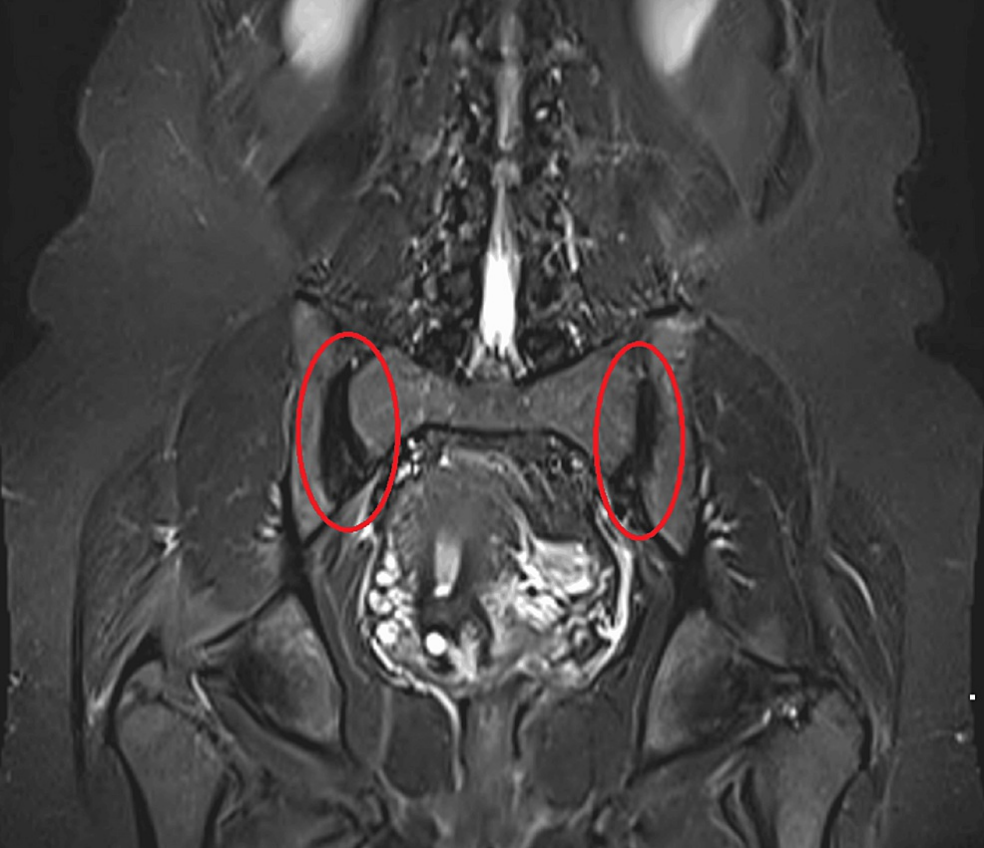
MRI showing focal sclerosis on the iliac aspect of both sacroiliac joints MRI: magnetic resonance imaging
